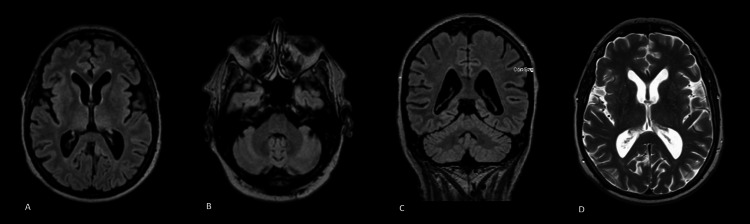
Brain MRI (Magnetic Resonance Imaging) study A- MRI axial FLAIR image: there are no focal areas of abnormal signal intensity or lesions identified. Both gray and white matter structures appear within normal limits, with no evidence of other pathological findings; B- MRI axial FLAIR image cerebellum: There is no evidence of cerebellar atrophy or other pathological findings;  C- MRI coronal FLAIR image: prominence of the cerebrospinal fluid (CSF) spaces and ventricular system, indicating increased CSF circulation pathways and a slight reduction in global brain volume, without clear lobar predominance; D- MRI axial T2-Weighted image: did not reveal any notable changes or abnormalities.
